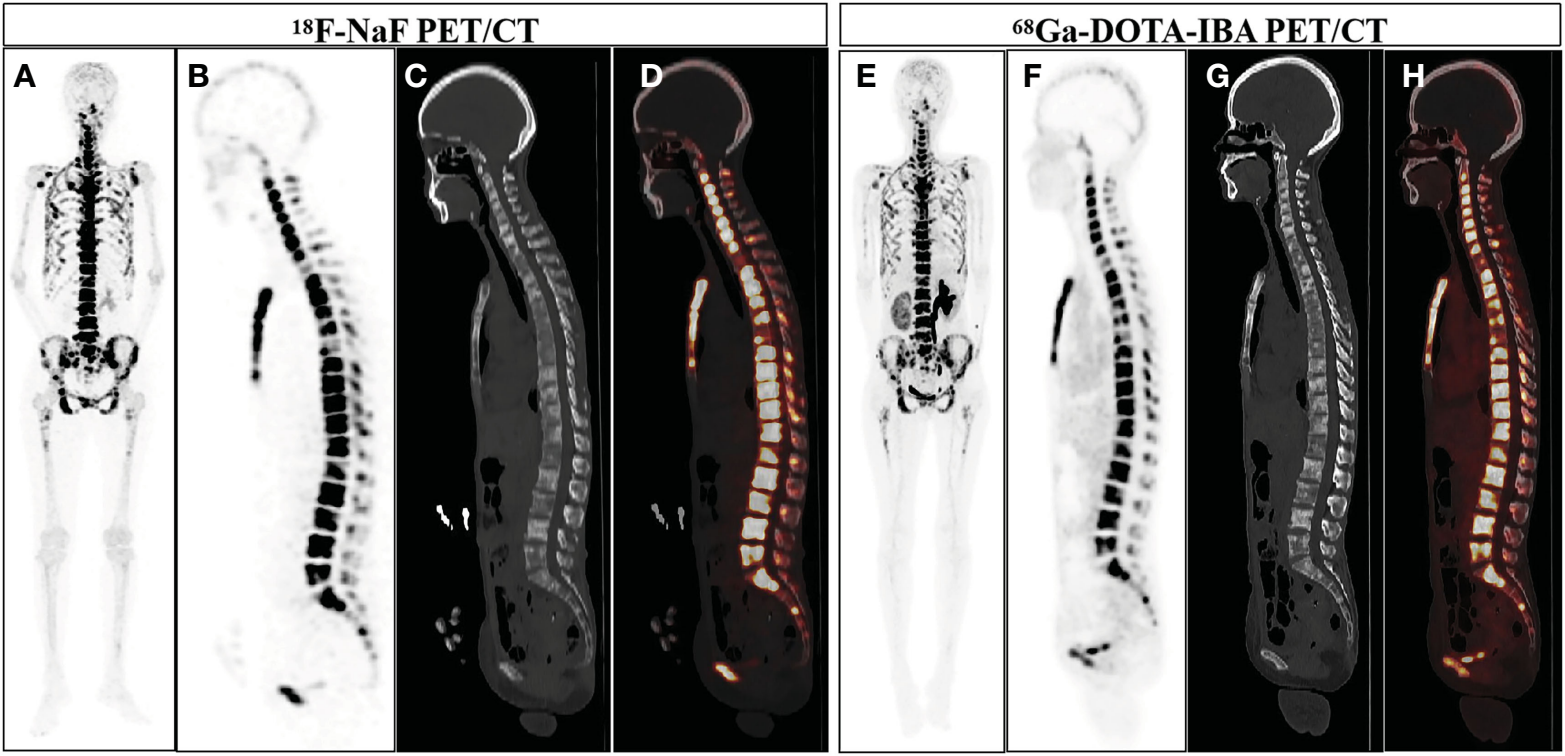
A 52-year-old woman who underwent gastric cancer surgery underwent ^18^F-NaF PET/CT examination for follow-up. The MIP image of ^18^F-NaF PET/CT (A) showed increased uptake of imaging agents in multiple skeletal areas throughout the body, and the sagittal views (B: PET image; C: CT scan; D: PET/CT fused image) more intuitively displayed bone destruction and increased uptake of imaging agents in multiple skeletal areas (SUVmax 76.5). ^68^Ga-DOTA-IBA PET/CT MIP (E) and the sagittal views (F: PET image; G: CT scan; H: PET/CT fused image) also showed increased uptake of bone imaging agents in multiple locations throughout the body (SUVmax 21.4).
